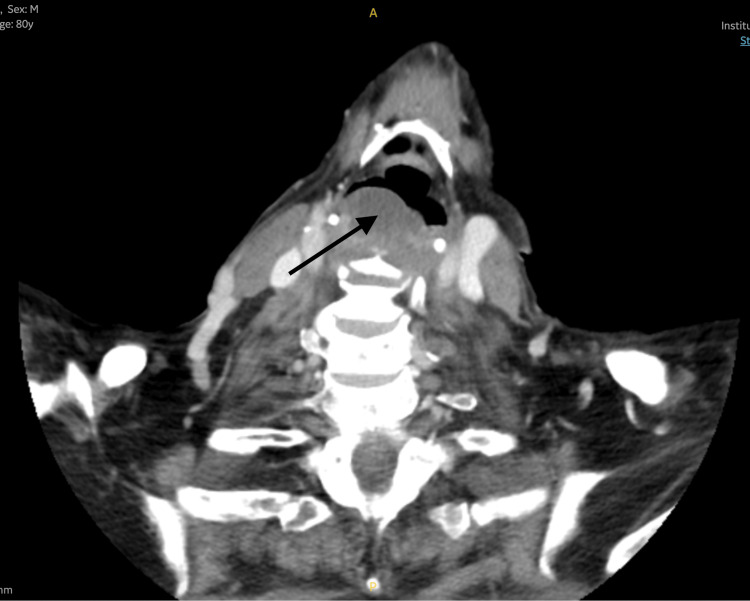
Axial view of the CT of the neck confirming a cystic lesion (black arrow) on the right side of the hypopharynx displacing the airway medially
